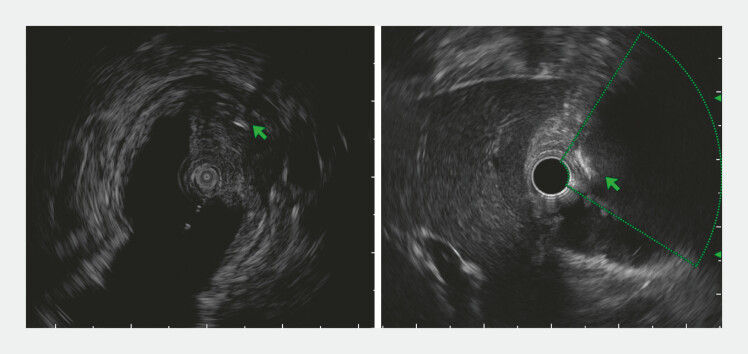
EUS was used to locate the striped hyperechoic linear foreign body (green arrow). EUS, endoscopic ultrasound.
